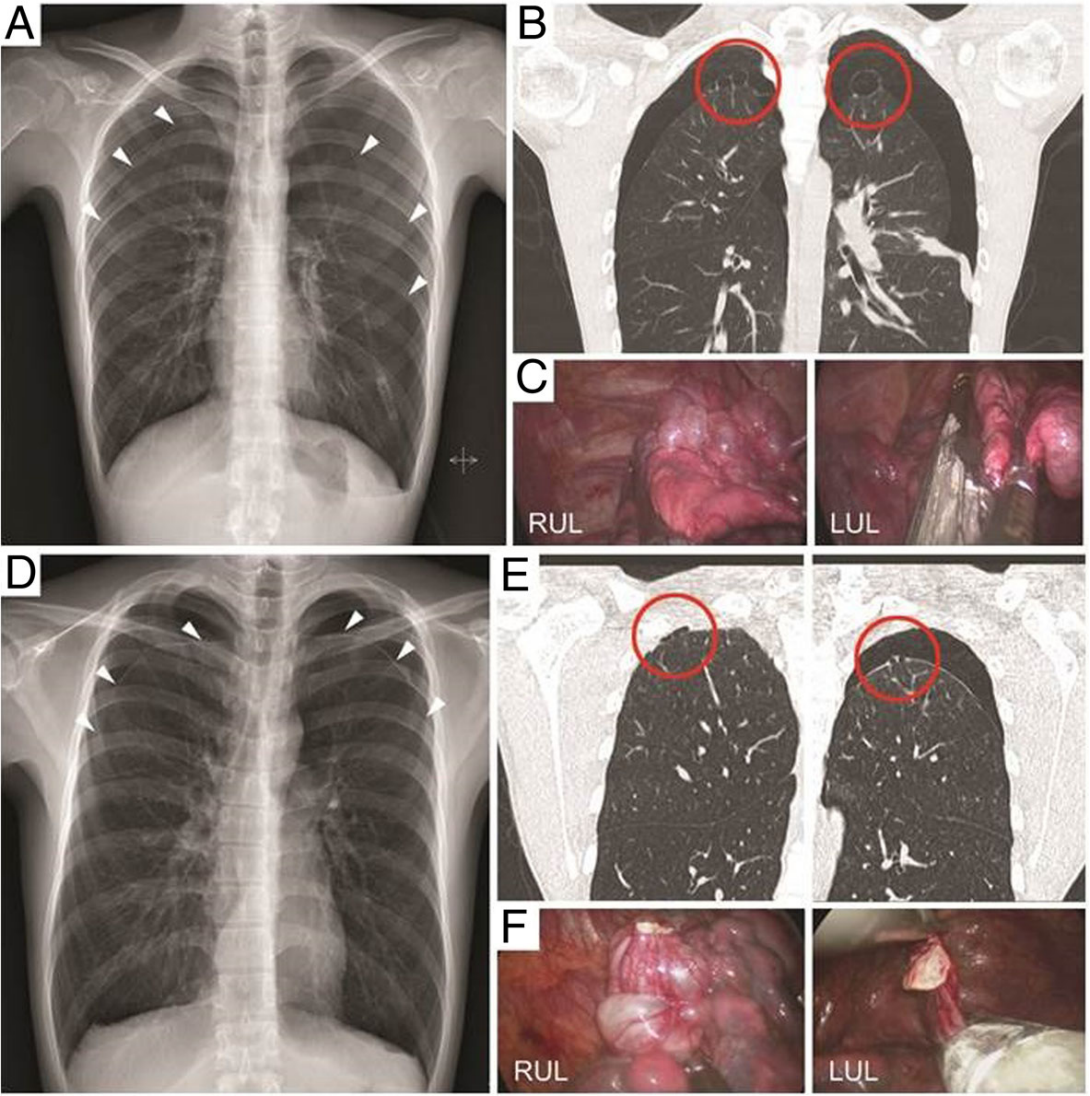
a, d Chest x-ray shows bilateral spontaneous pneumothorax of case 1 and 2 (*white arrow head*). b, e Chest tomography image shows multiple, variable-sized apical bullae on both lung apices (*red circle*). c, f Wedge resection of bilateral bullae lesions using endostaplers under 5-mm, 30° thoracoscopic view
